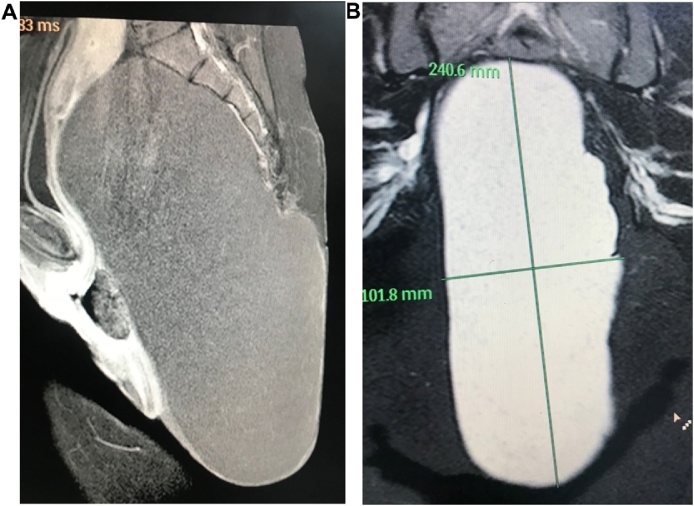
(A): MRI of the pelvis showing cyst filled with faint calcifications with clear margins except over the lower coccyx compressing the pelvic structures anteriorly and superiorly. (B): MRI showing dimensions of the cyst and relations with pelvic structures.
